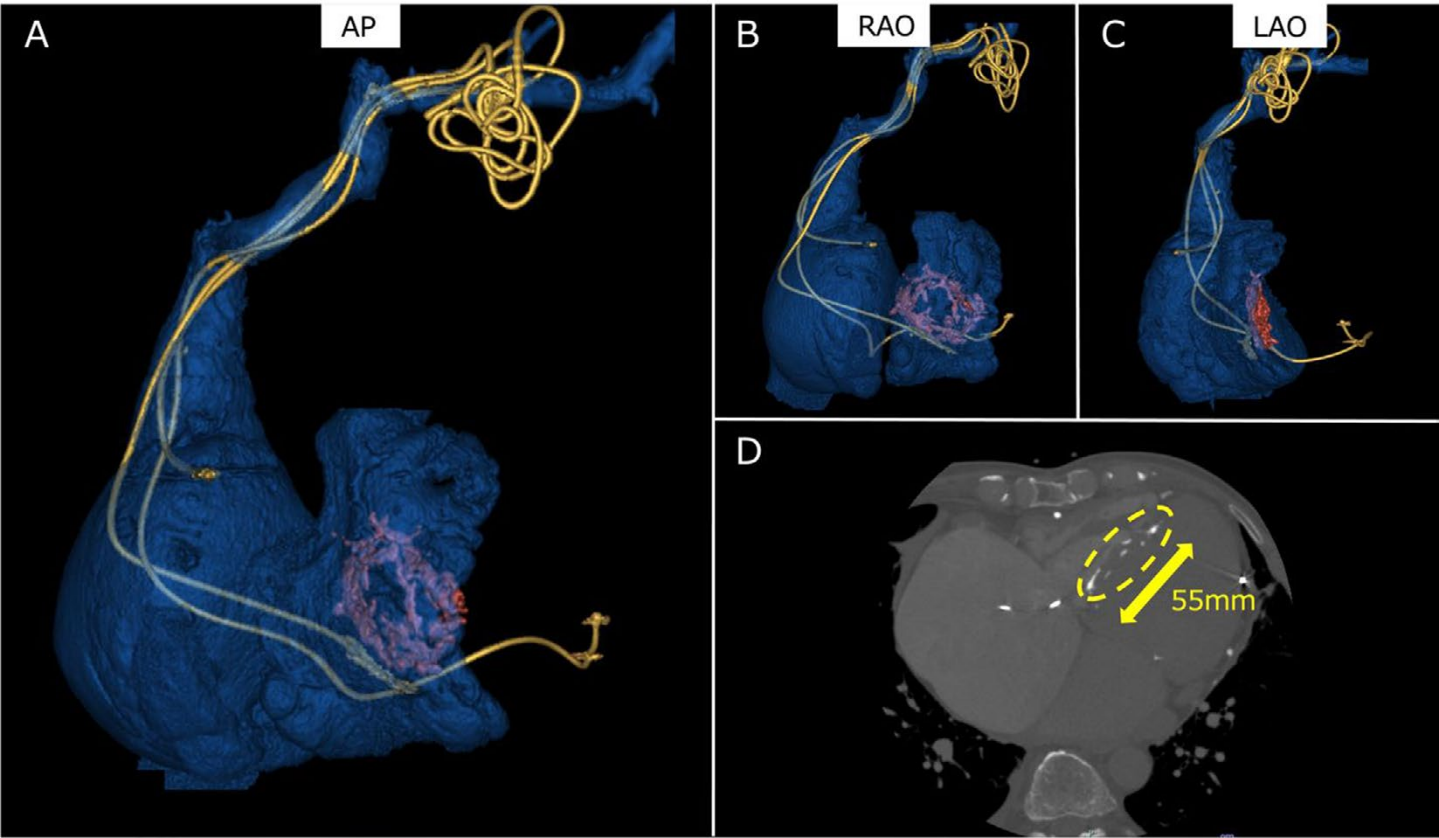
(A) Anteroposterior (AP), (B) right anterior oblique (RAO), and (C) left anterior oblique (LAO) views of 3D reconstructed CT images showing the course of the lead; the orange‐colored area represents the VSD patch. (D) Axial CT image demonstrating the VSD patch with surrounding calcification, indicated by the yellow dotted line. The maximum diameter of the patch is 55 mm.
